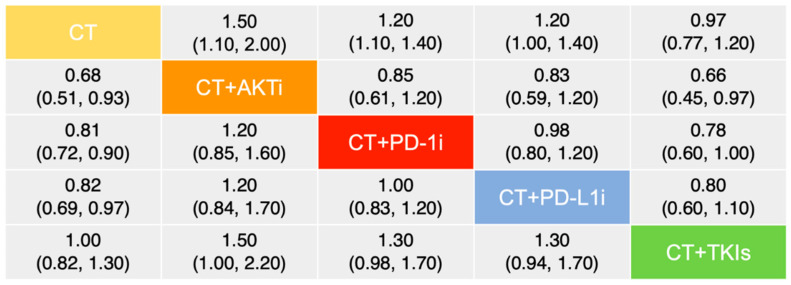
Comparative analysis of progression-free survival among selected therapies. Data are presented as hazard ratio and 95% credible interval. The upper right values display the top treatment compared to the bottom treatment. The lower left values demonstrate the converse comparisons. Abbreviation: AKTi, Protein kinase B inhibitor; CT, Chemotherapy; PD-1i, Programmed cell death protein 1 inhibitor; PD-L1i, Programmed cell death ligand 1 inhibitor; TKIs, Tyrosine kinase inhibitors.
